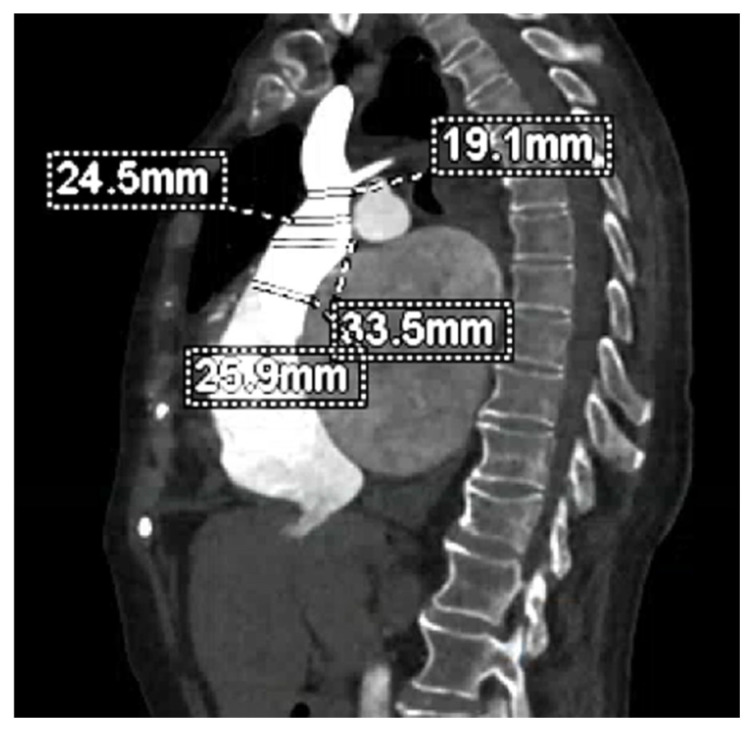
CT-based sizing of the superior vena cava. Cardiac CT image showing superior vena cava sizing in the context of pre-procedural planning for heterotopic caval valve implantation in a patient with torrential tricuspid regurgitation.
